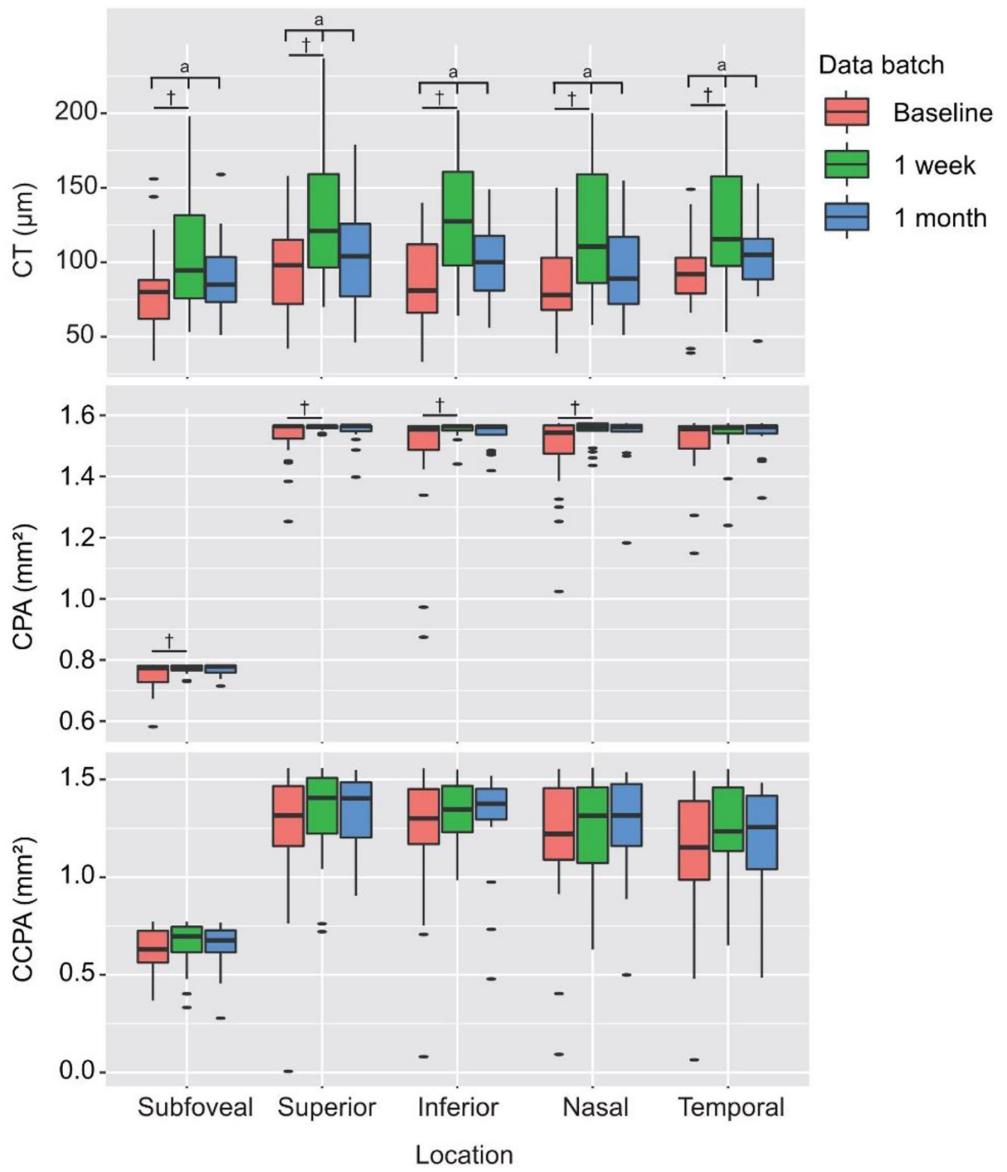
Preoperative and postoperative measurements of CT, CPA, and CCPA of the center subfield and parafovea (superior, inferior, nasal, and temporal subfields). ^a^Statistically significant at *P* < 0.05 by RMANOVA, ^†^Statistically significant at *P* < 0.05 by multiple comparisons (LSD *post-hoc* test) vs. the baseline.
